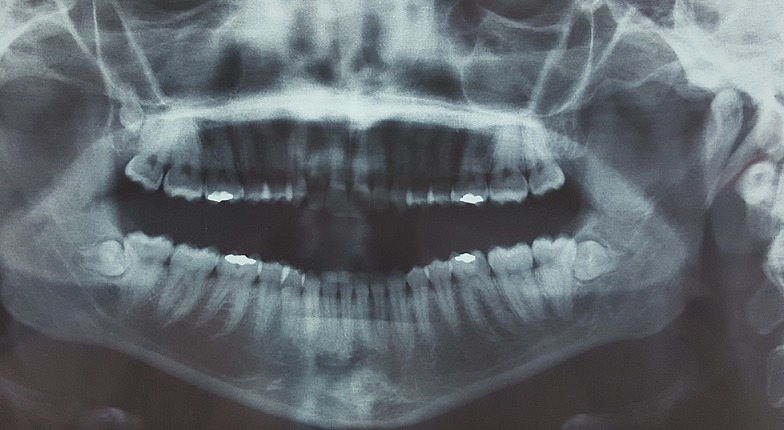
Orthopantomogram showing the presence of supernumerary teeth behind 18, 48 and 38
